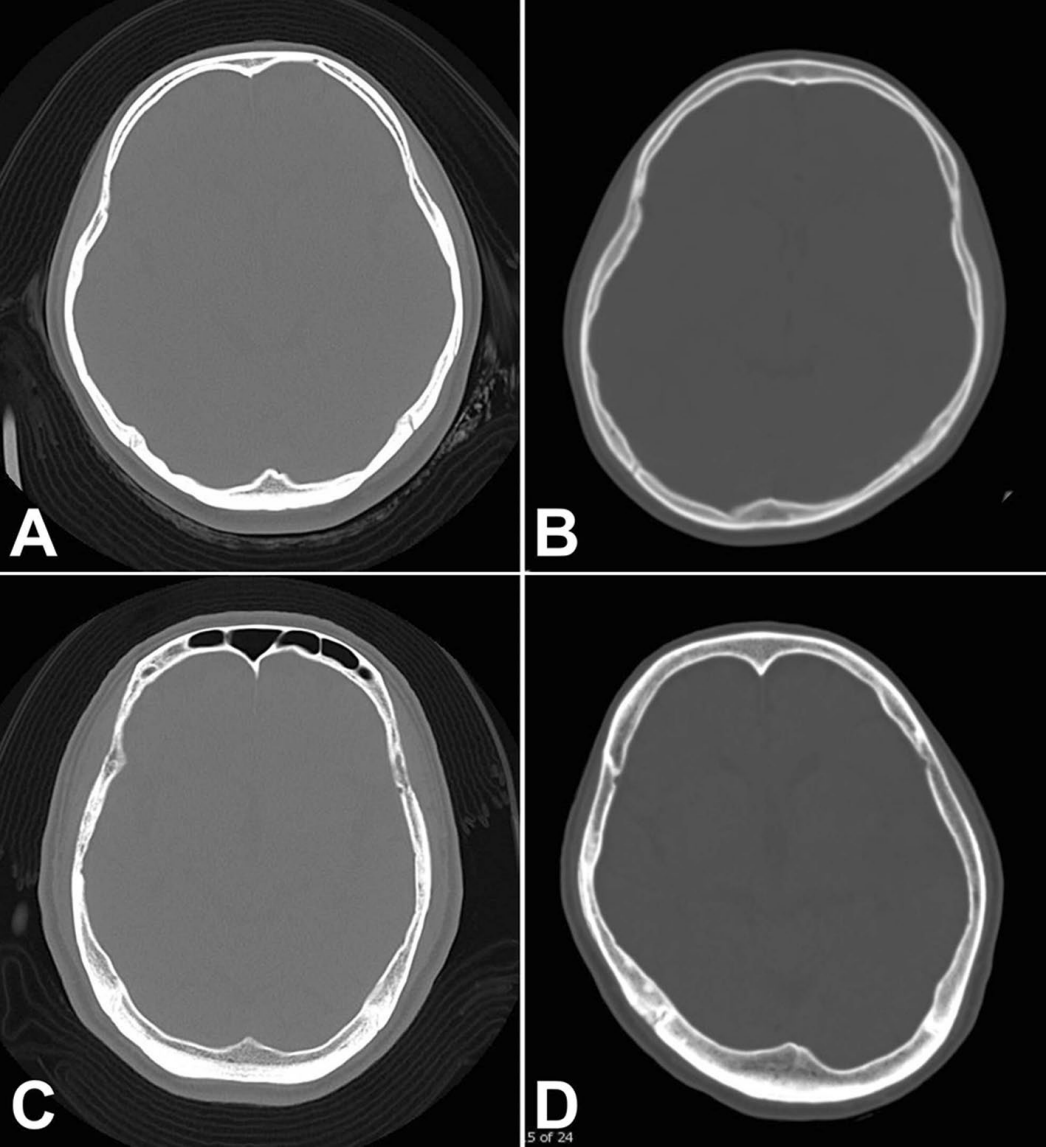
Representative computed tomography scan findings. An axial computed tomography scan of the head with a bone window from head injury (A), moyamoya disease (B), cervical internal carotid artery stenosis (C), and depression (D).
